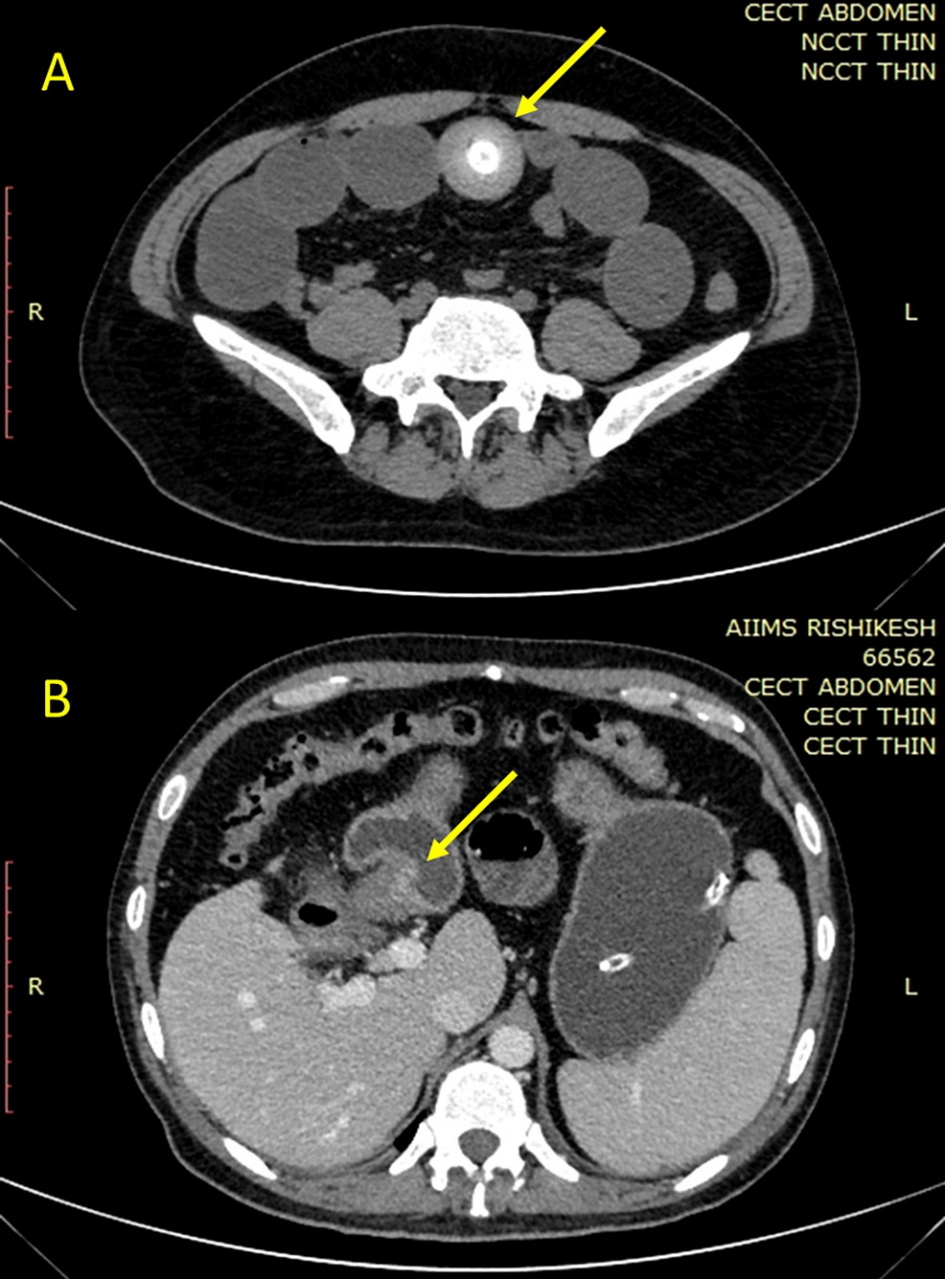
CT scan of abdomen showing (A) dilated small bowel loops with lamellated hyperdense calculus ~17x15mm in lumen of distal jejunal loops at the transition point with collapsed distal bowel, and (B) thickened gallbladder wall with fistulous communication between the gallbladder and the duodenum
